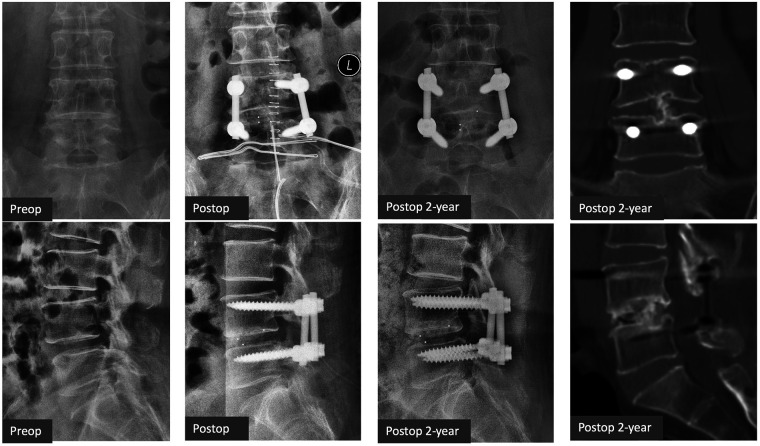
Case example of TLIF. A 56-year-old female patient with L4/5 degenerative spondylolisthesis had neurological claudication and low back pain (ODI 50%, VAS-Leg 5, VAS-Back 5). The symptoms were subsided after TLIF (ODI 16%, VAS-Leg 1, VAS-Back 2) at 2 years. Grade 2 fusion (patchy bone bridging) on CT scans was achieved at 2 years. TLIF, transforaminal lumbar interbody fusion; ODI, Oswestry disability index; VAS, visual analog scale.
